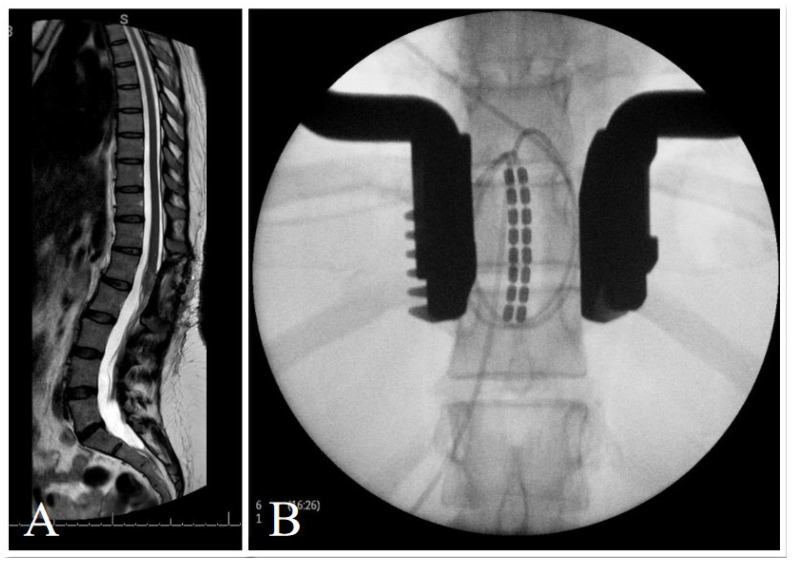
Retrograde paddle insertion at T9-10 for a case of post-ependymoma resection neuropathic pain in a 49-year-old female. Pre-operative MRI (A) and intra-operative X-ray (B). Boston Microburst was employed with a best anatomical location of T9.
